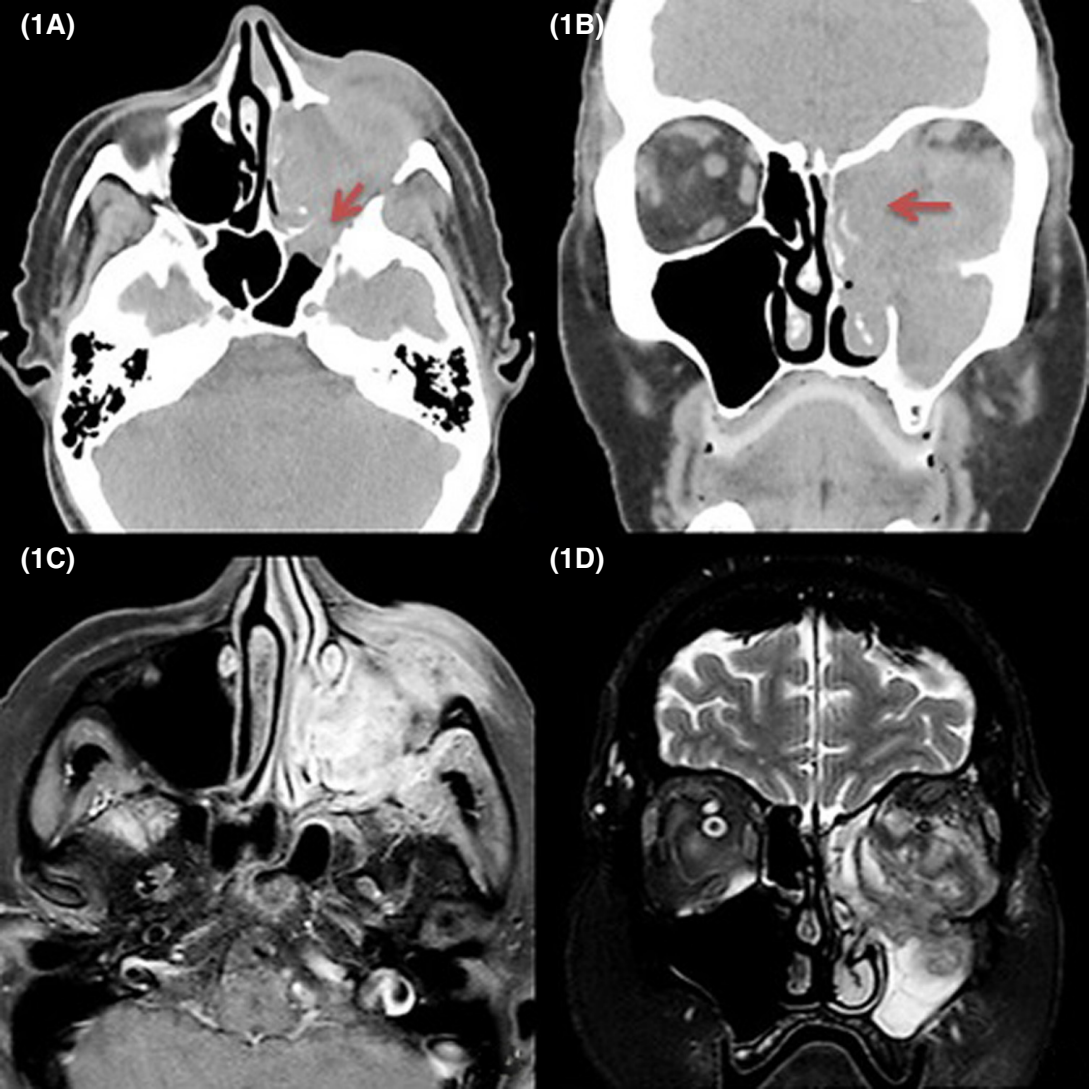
(A and B) Contrast‐enhanced, high‐resolution CT in axial and coronal plane images, respectively, showing a lesion with soft‐tissue density causing bone discontinuity of the maxilla, septal, sphenoid, ethmoidal, and pterygopalatine fossa (arrows). (C) T1‐weighted postcontrast MRI axial plane image showing a lesion with heterogeneous enhance affecting the left maxillary sinus, extending to the face. (D) T2‐weighted coronal plane image showing a heterogeneous mass in the region of the maxillary sinus, extending to the orbital region.
